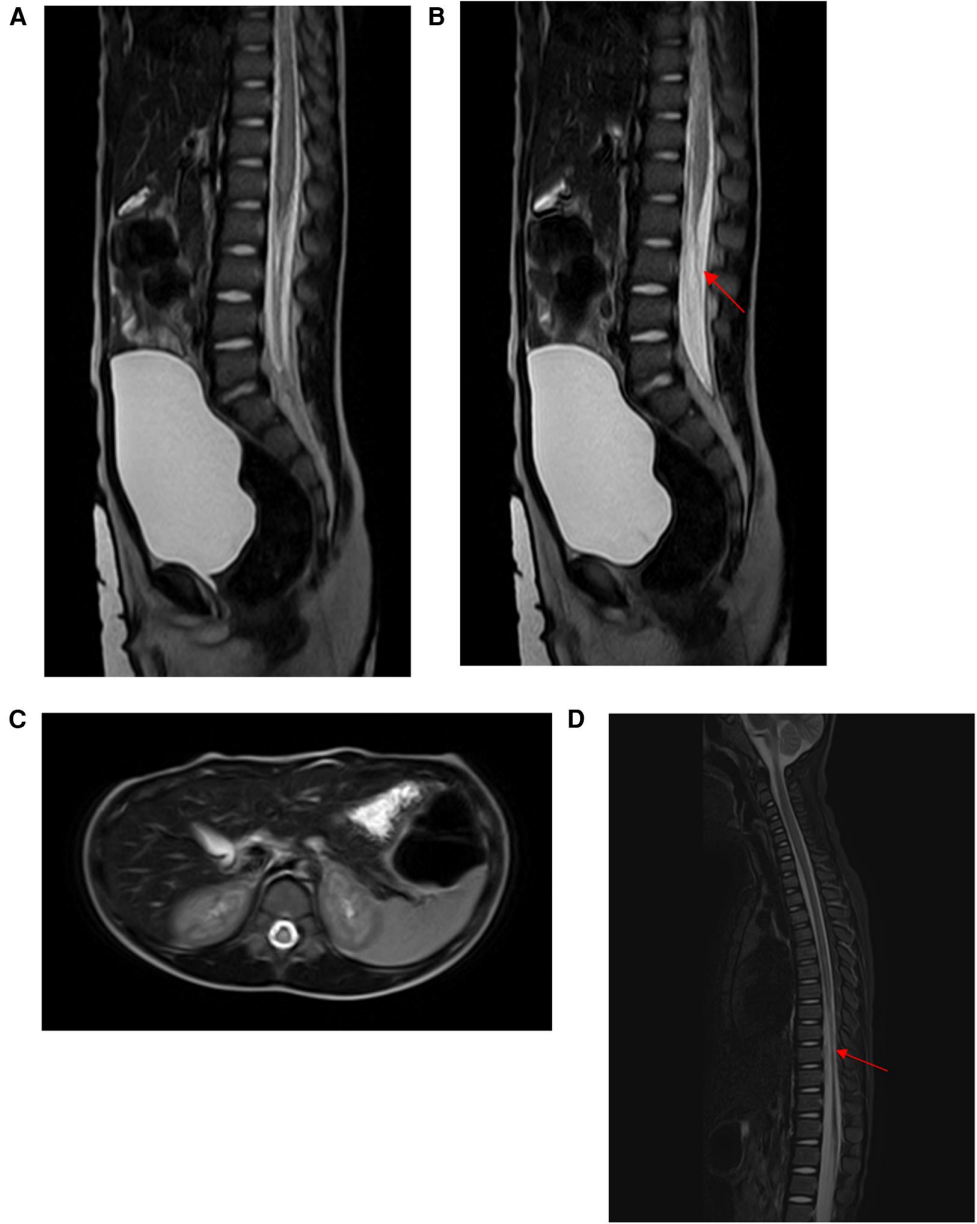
(A,C) MRI revealed significant spinal cord edema. (B) MRI revealed the filum terminale was thickened and deviated dorsally, suggesting the possibility of tight filum terminale. (D) Five months postoperatively, MRI revealed spinal cord atrophy.
